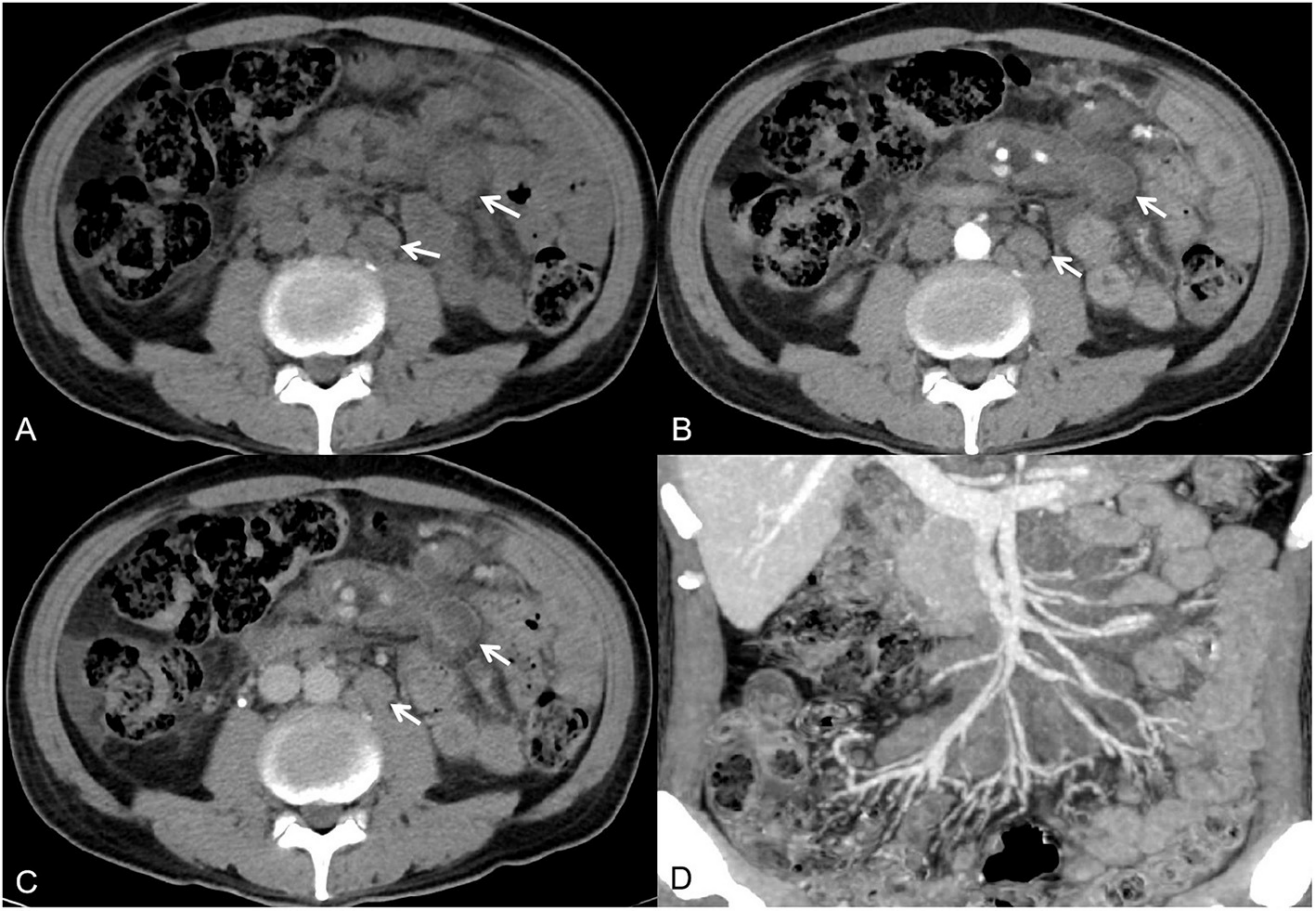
A 54-year-old man had recurrent fever and malaise for 1 month. Axial non-contrast-enhanced CT imaging showed multiple enlarged lymph nodes in the mesentery and retroperitoneum [(A), white arrow]. Axial contrast-enhanced CT images showed lymph nodes with rim or homogeneous low enhancement [(B,C), white arrow]. The maximum intensity projection image showed that the surrounding blood vessels were not invaded (D).
